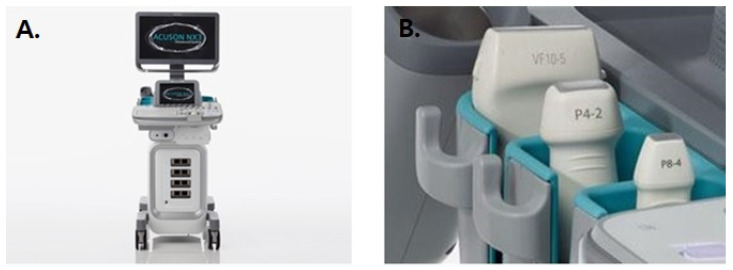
Measurement device (ACUSON NX3 Elite. SIEMENS-Healthineers. Germany). (A) Arterial view: ultrasonography device used for the study. (B) Probe for measuring Doppler ultrasound.
